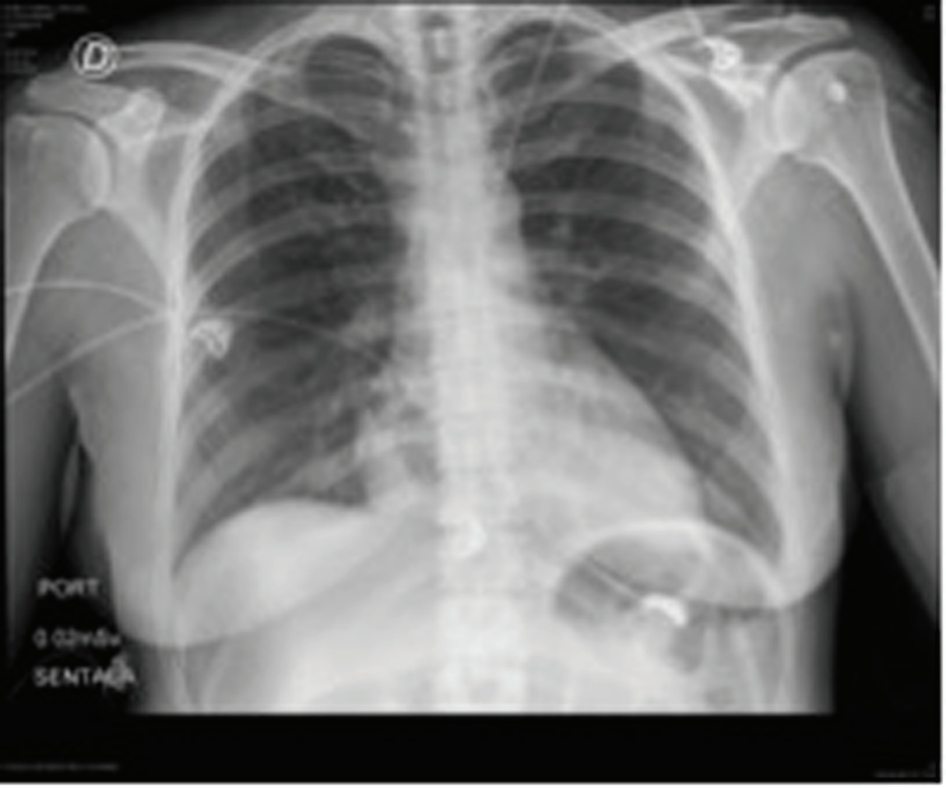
Patient’s initial chest X-rays.
